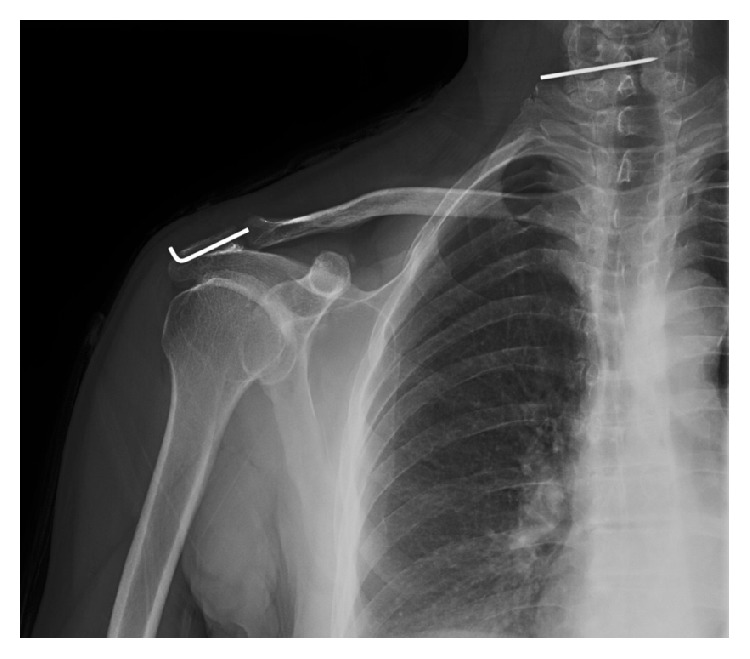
Radiographic image of migration of a broken K-wire used in the fixation of the right AC joint dislocation.
